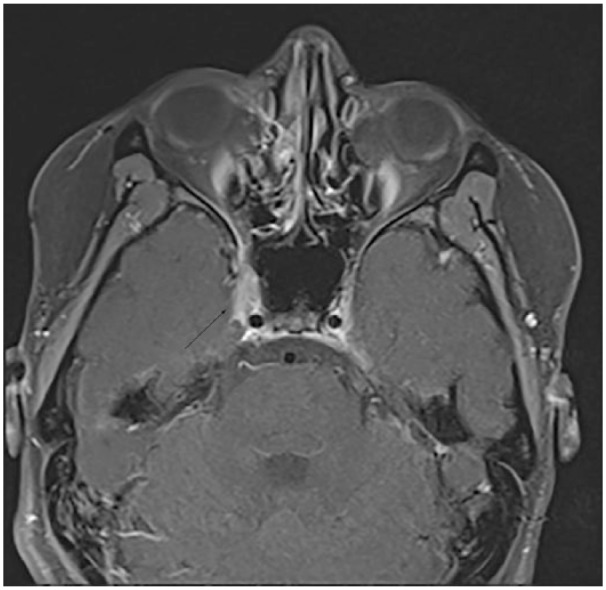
MRI of the orbits shows an asymmetric mass-like enhancement of right cavernous sinus region depicted by the black arrow.
